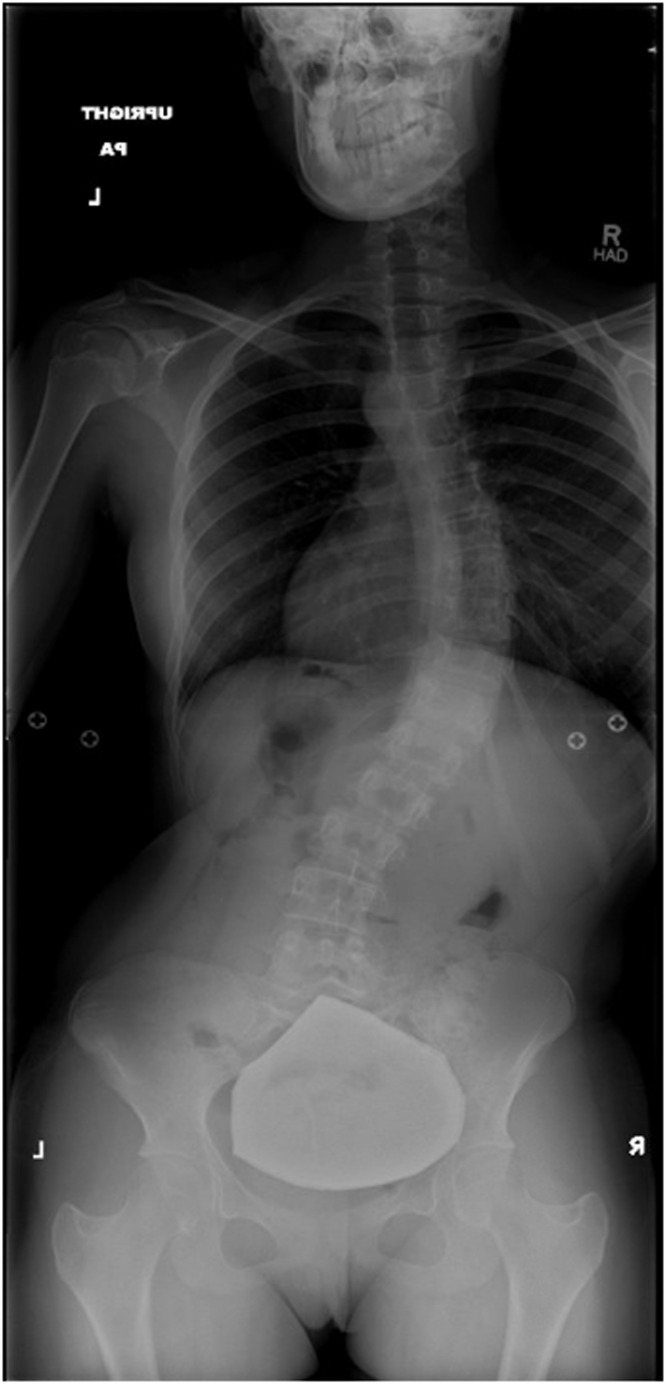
Anteroposterior plain radiograph film showing a 49° dextroscoliosis of the thoracolumbar spine centered at T9/T10 and a 30° levoscoliosis of the lower lumbar spine centered at L4.
